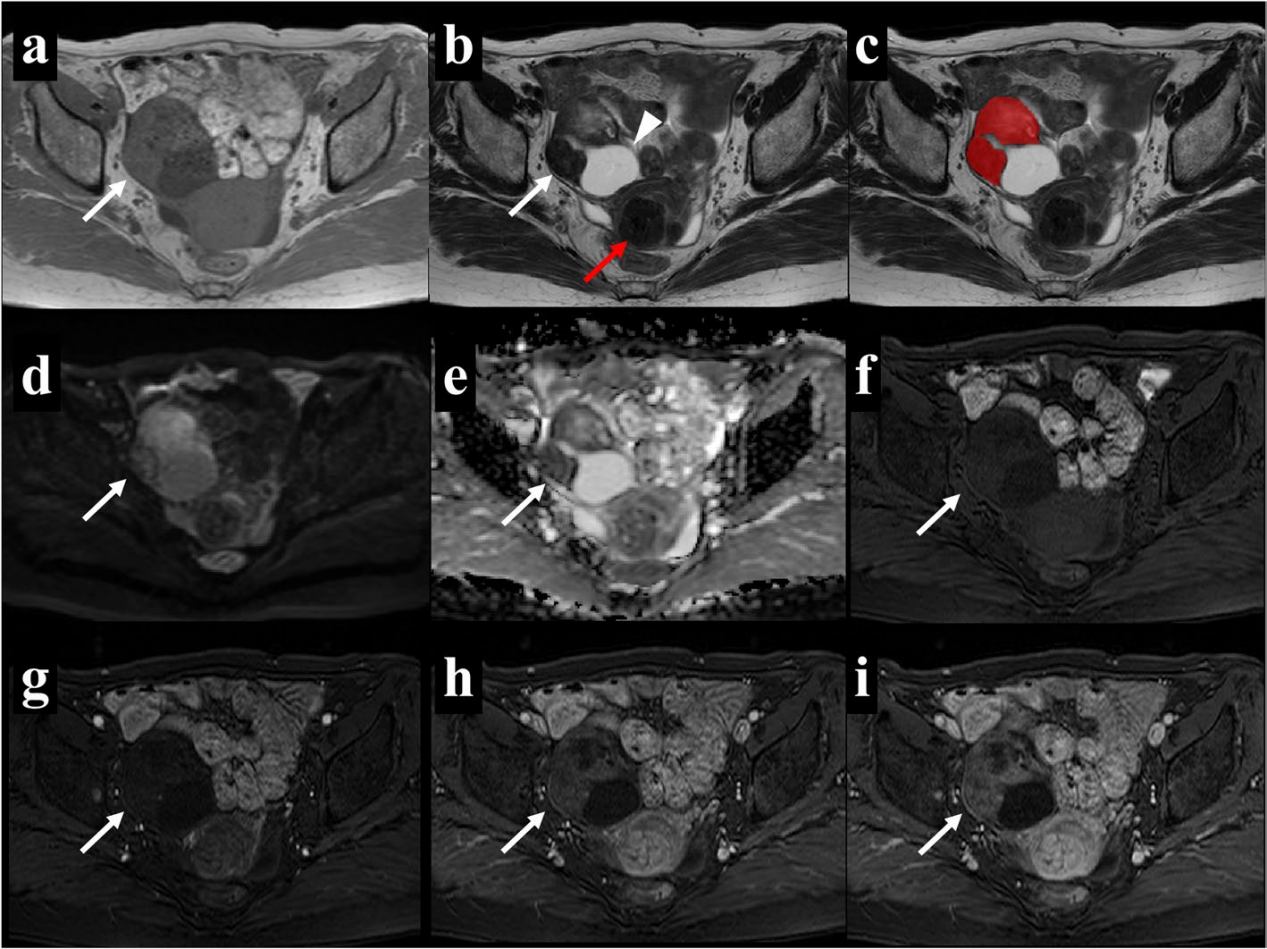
A 66-year-old woman with right ovarian fibrothecoma. Axial T1WI (a) showed an isointense solid mass and axial T2WI (b) showed a heterogeneous solid mass with a cystic change in the dorsal part (arrowhead). Uterine fibroids were present (red arrow). The region of interest (ROI) in the texture analysis (c) was delineated to contain the outline borders of the solid region of the tumor, and the cystic region was avoided as much as possible. Axial DWI (d) showed a hyperintense solid area, and the apparent diffusion coefficient (ADC) map (e) showed that the average ADC value of this area was 1.31 × 10^−3^ mm^2^/s. In multiphasic contrast-enhanced fat-suppressed T1-weighted MRI (f-i), the mass showed minimal enhancement in the arterial and venous periods (g and h, respectively) as well as heterogeneous mild enhancement in the delayed period (i)
